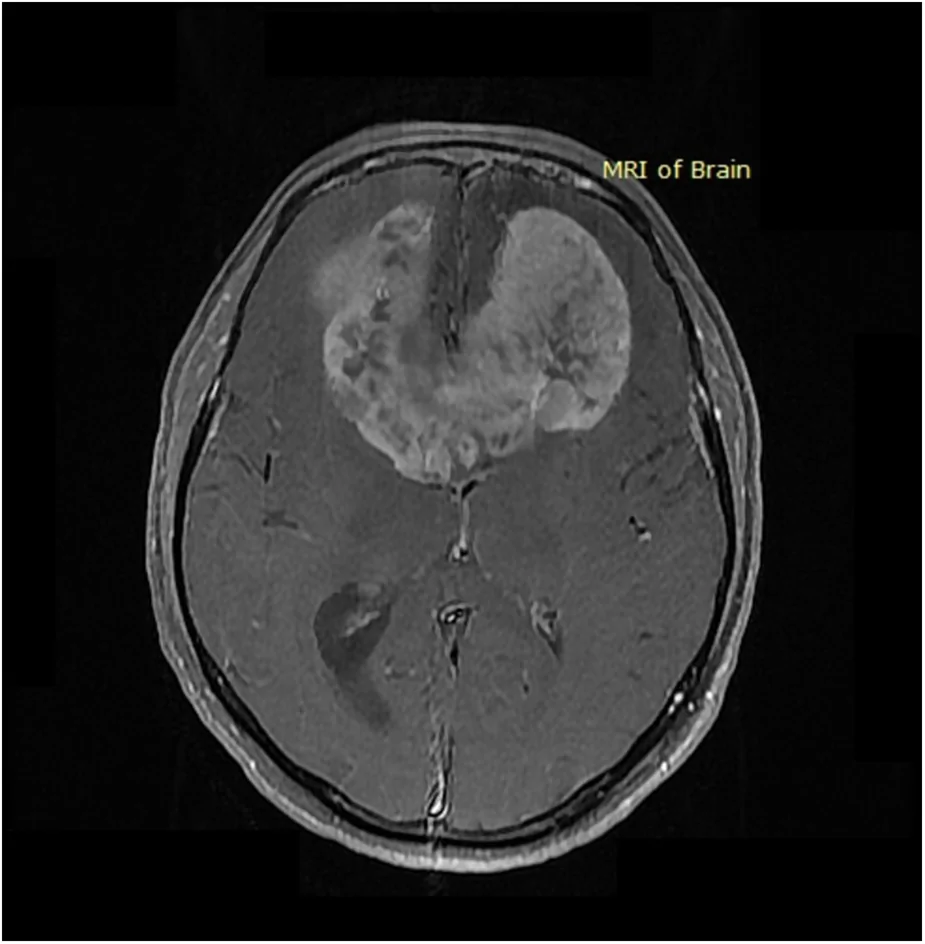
Butterfly glioblastoma. Axial T1-weighted post-contrast MRI demonstrates a heterogeneously enhancing “butterfly” lesion centered in the frontal lobes, crossing the genu of the corpus callosum. The lesion exhibits diffuse heterogeneous enhancement and central necrosis, imaging features characteristic of a high-grade glioma.
